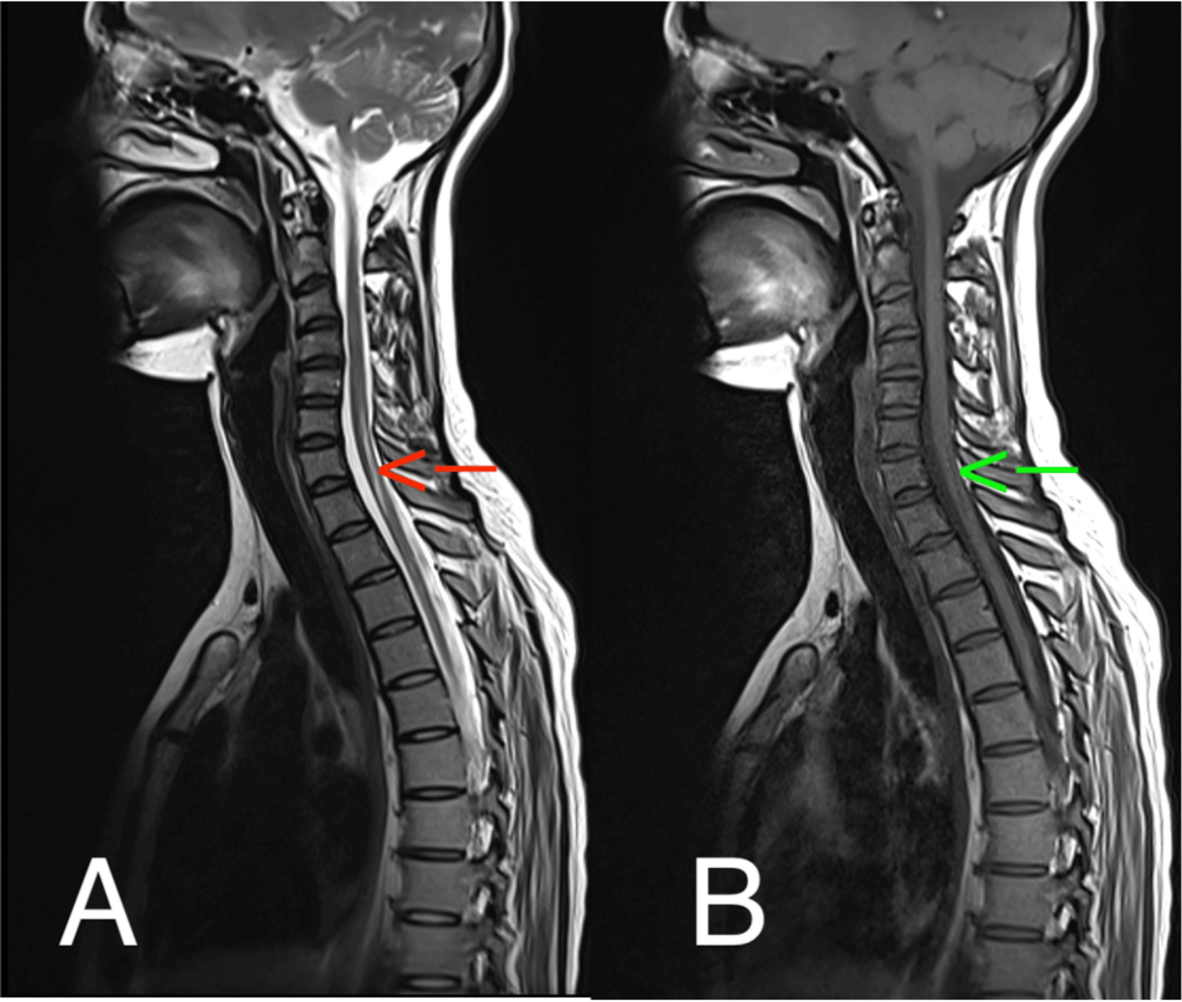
Thoracic spinal MRI demonstrating LETM (a) Sagittal T2-weighted (T2W) image shows an intramedullary hypersignal (red arrow) extending from T1 to T4 vertebral segments, consistent with edema or inflammation. (b) Corresponding sagittal T1-weighted (T1W) image displays an intramedullary hyposignal (green arrow) at the same level, reinforcing the diagnosis of myelitis. LETM: Longitudinally extensive transverse myelitis.
